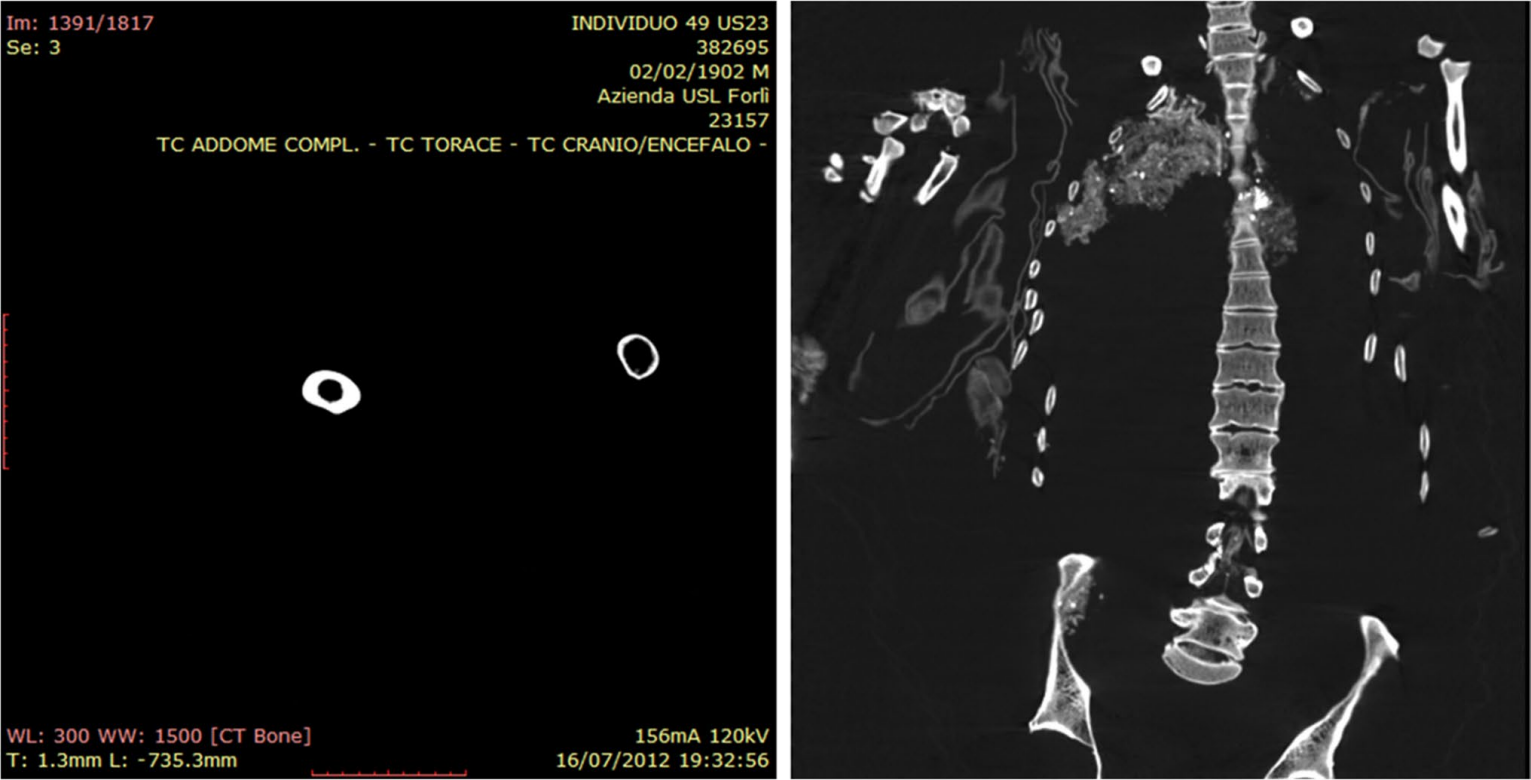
Left: cortical thickness differences of the femoral mid-shaft in axial view from the CT scan. Right: sagittal view of the vertebral column with numerous Schmorl’s nodes visible on the T8-T12 spinal segment
